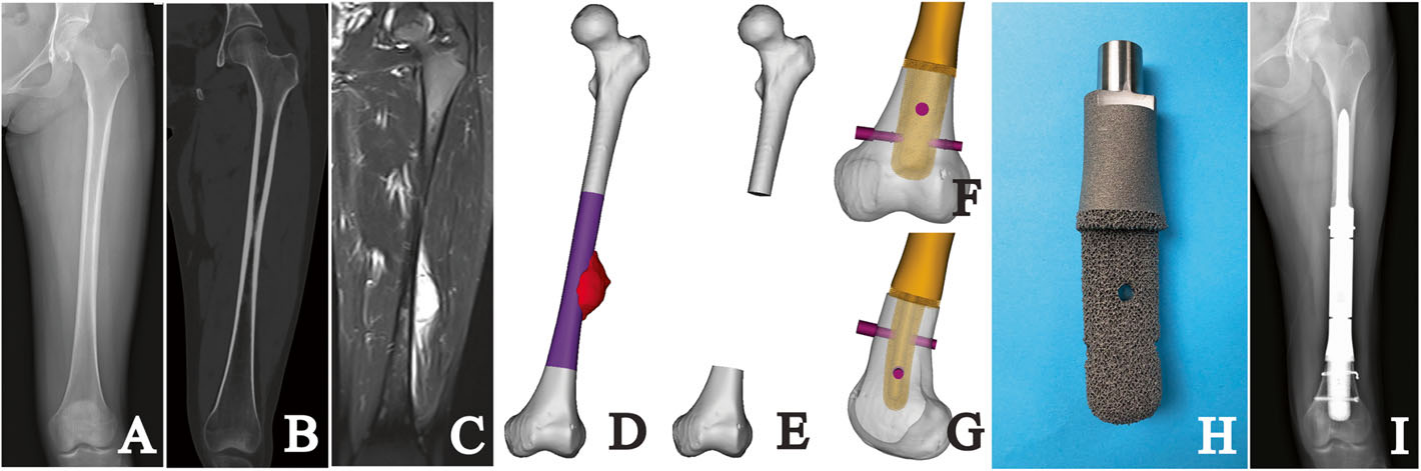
A The AP radiographic view shows an Ewing sarcoma of the diaphyseal left femur (patient 1). The B CT and C MR images show extension in the surrounding tissues. The virtual D tumor model and E defect model in Mimics software is shown. The F AP view and G lateral view of stem design in distal femoral stump are shown. H The photograph shows the porous structure and whole 3D-printed custom-made stem fabricated with electronic beam melting technique. I The AP radiograph at the latest follow-up is shown
